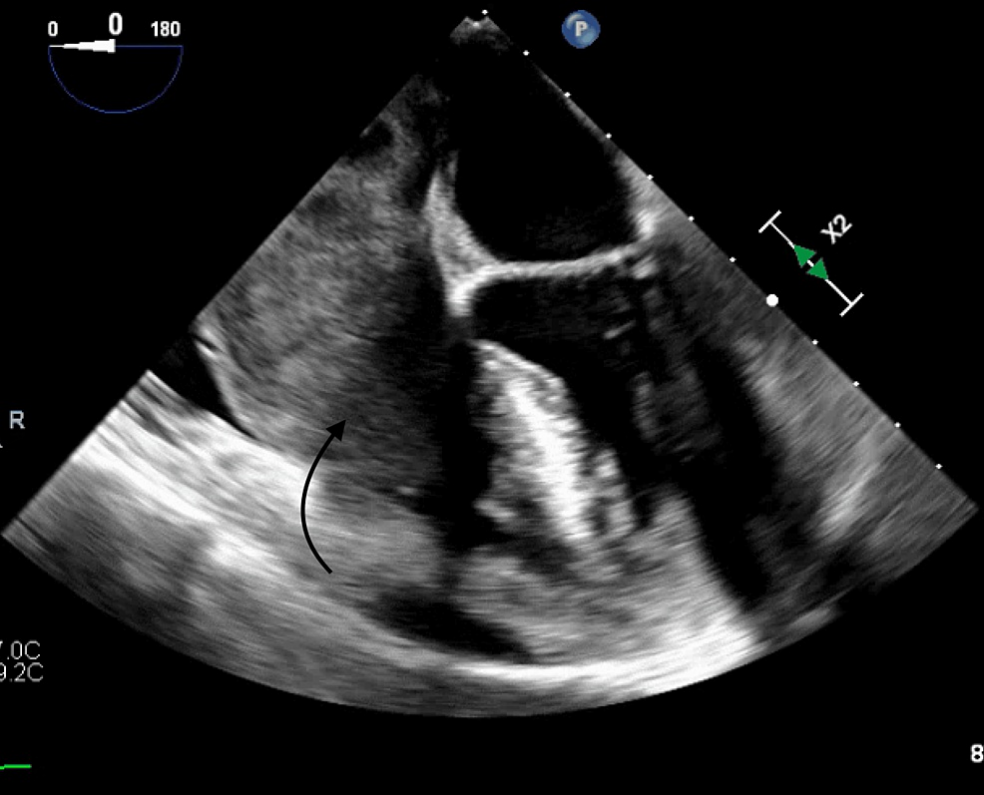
Transesophageal echocardiogram showing right atrium mass extending into the right ventricle.
